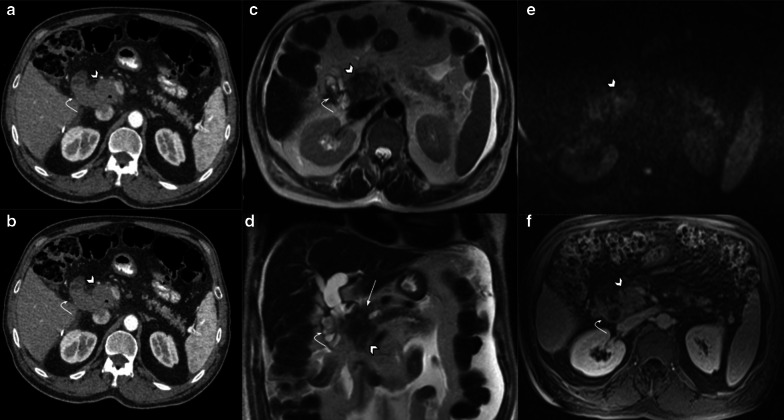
Collapsed loop vs tumor recurrence. The MRI role. 58-year-old male patient during routine follow-up after pancreaticoduodenectomy due to pancreatic adenocarcinoma. (a, b) Control CT scan. Well-defined enhancing soft tissue image (arrowhead) between the afferent loop (curved arrow) and the pancreaticojejunostomy. Collapsed loop versus tumor recurrence were suggested as possible diagnosis, so MRI was indicated. (c, d, e, f) MRI performed three days later revealed a heterogeneous ill-defined mass located between afferent loop (curved arrow) and pancreaticojejunostomy (straight arrow in d). Note the better tissue differentiation of MRI over CT, ruling out collapsed loop diagnosis. Restriction on DWI (e) and contrast enhancement (f) was also noted (arrowhead in e and f). Local recurrence was confirmed by PET-CT
